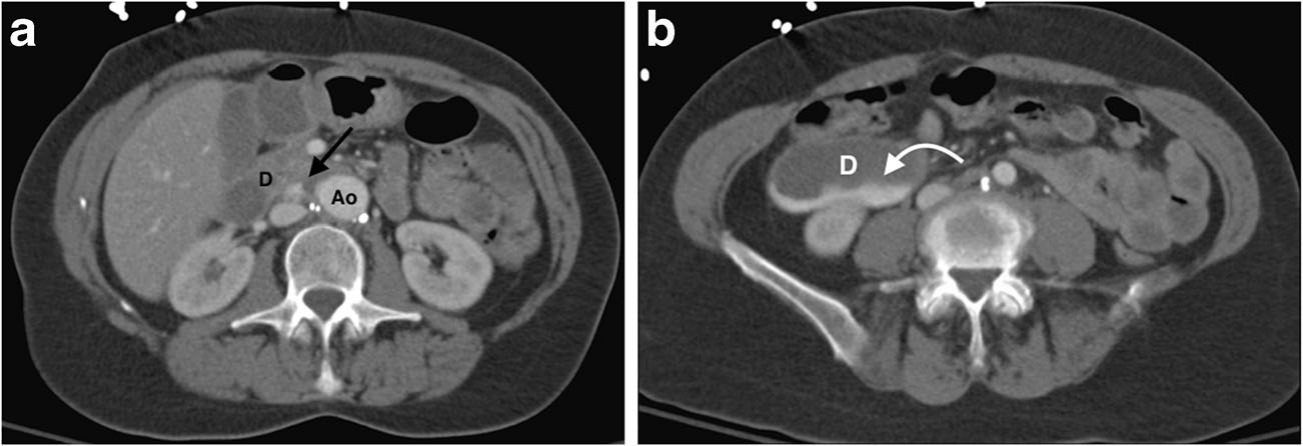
A 62-year-old woman with a history of a previous open abdominal aortic aneurysm graft repair presents with abdominal pain and emesis of brown particulate matter, and was referred for CT angiogram. (a, b) Axial slices of a CT scan of the abdomen acquired 70 s post-contrast demonstrates a fistula (*arrow*) between the aorta (Ao) and duodenum (d), with layering of high-density material in the duodenum (*curved arrow*). The patient underwent emergency surgery, but unfortunately died. Post mortem culture of the abdominal aorta graft grew gram-negative bacilli, likely *E. coli*
